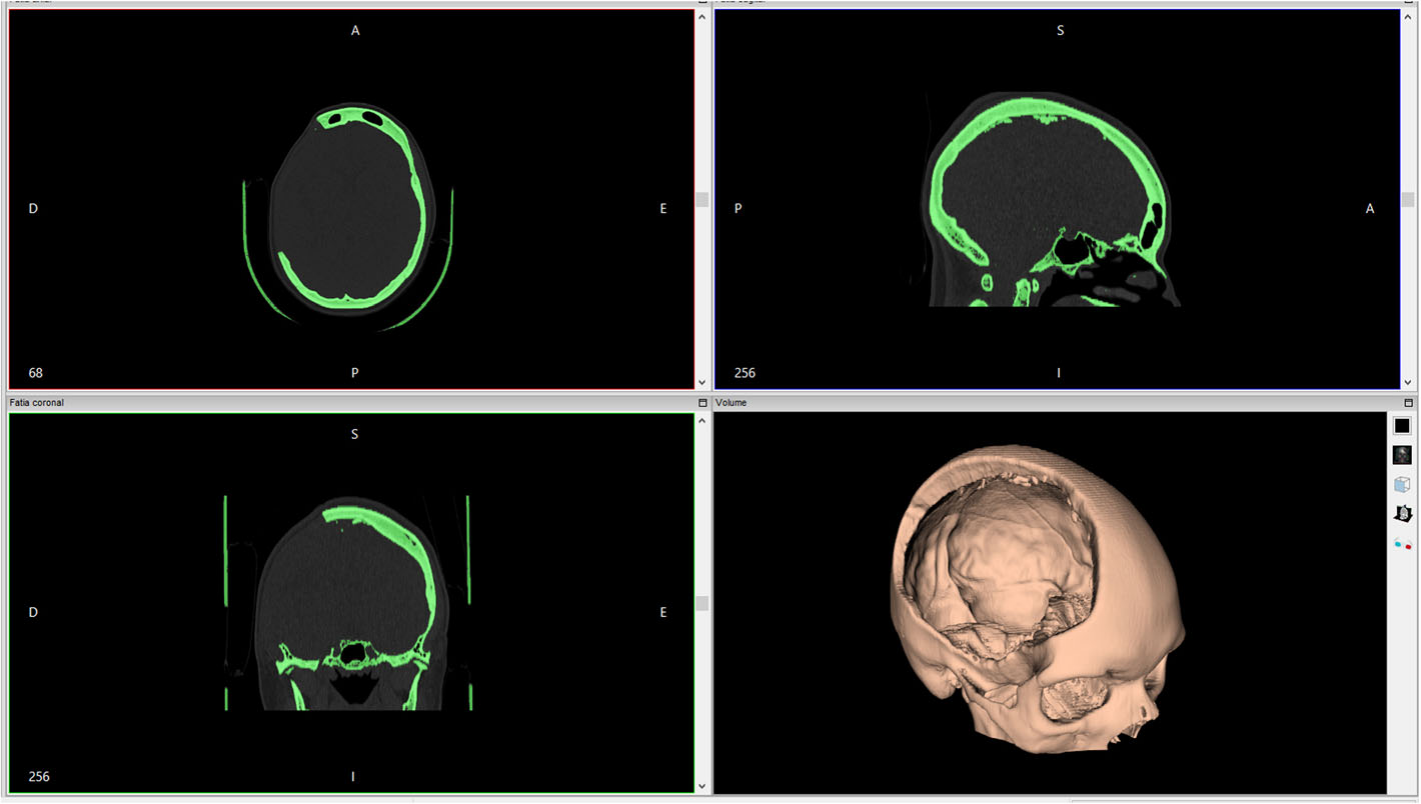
Three-dimensional rendering with computed tomography scan
